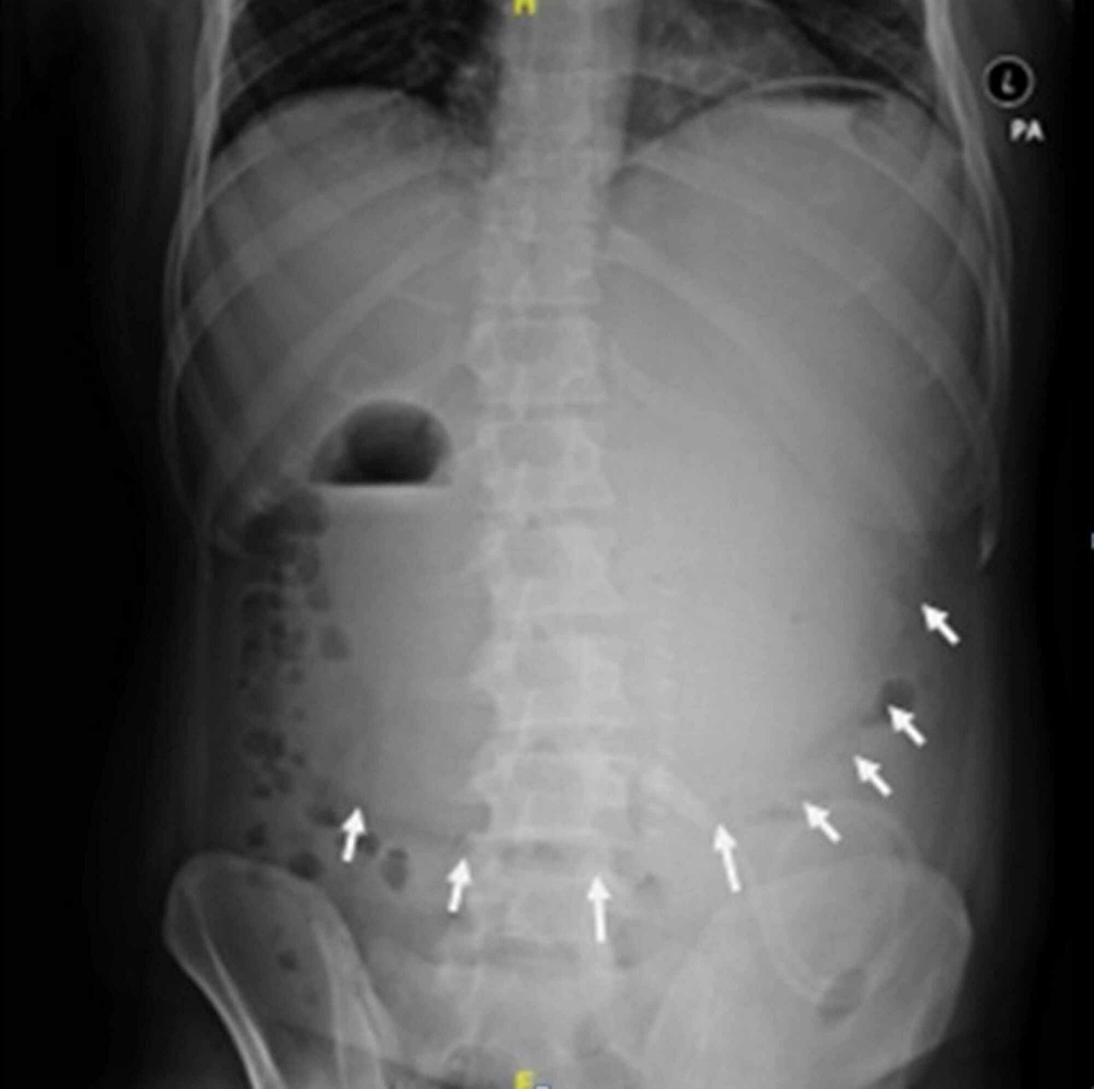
X-ray of the abdomen showing a dilated stomach (arrows are depicting greater curvature of the stomach).
